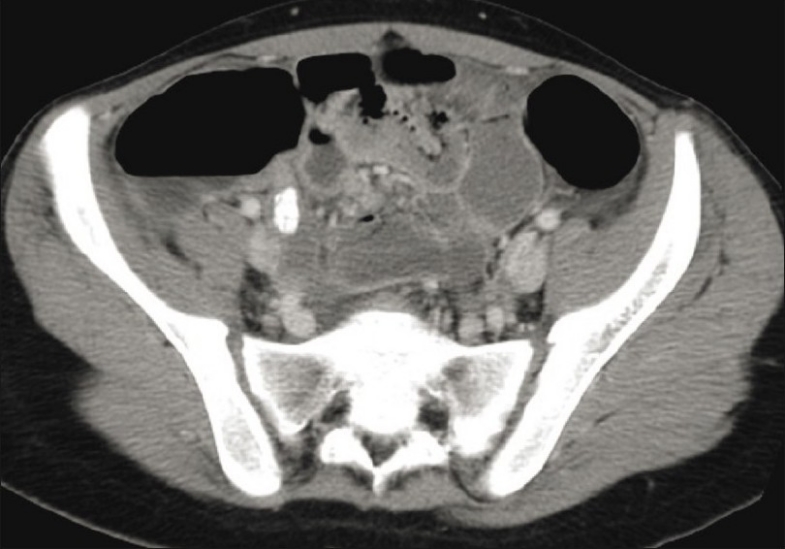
An 18 year-old female with right lower quadrant pain. CT scan with oral and IV contrast shows an appendicolith in an inflamed appendix
